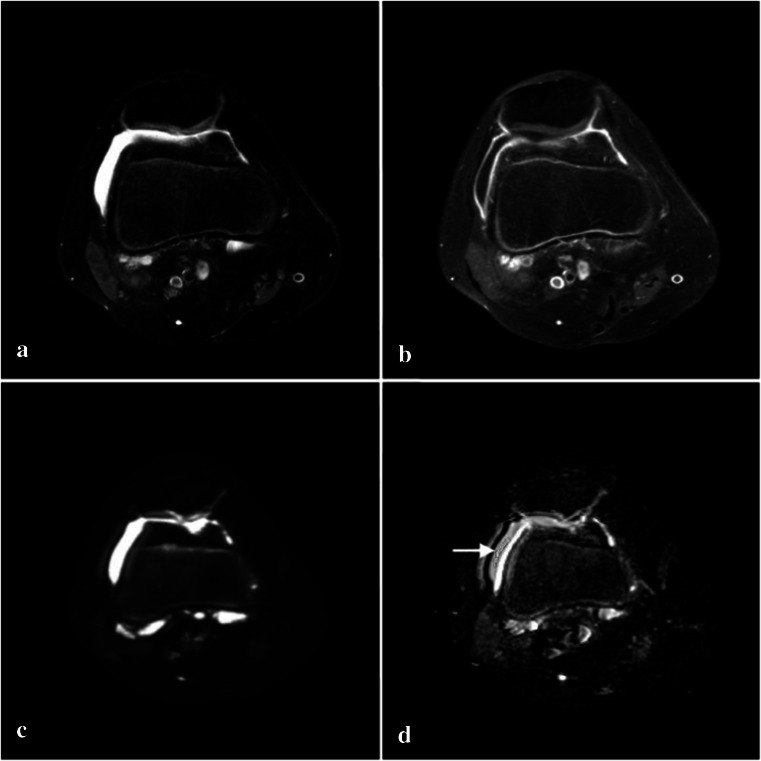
MRI of the knee of an 11-year-old female JIA patient. ROI selection of effusion on ADC_50–600_ map (*arrow points*
